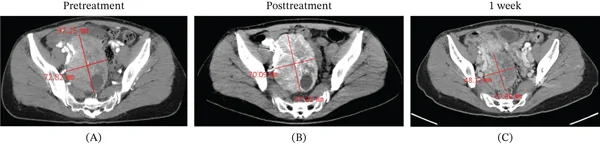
(A) Computed tomography (CT) image of the ovary in a patient with a Krukenberg tumor prior to treatment with docetaxel liposomes and sonodynamic therapy (SDT) using verteporfin liposomes. A large tumor is visible in the right ovary. (B) CT image obtained 1 day after the initial treatment shows no significant change. (C) CT image obtained 1 week after treatment demonstrates a marked reduction in tumor size.
